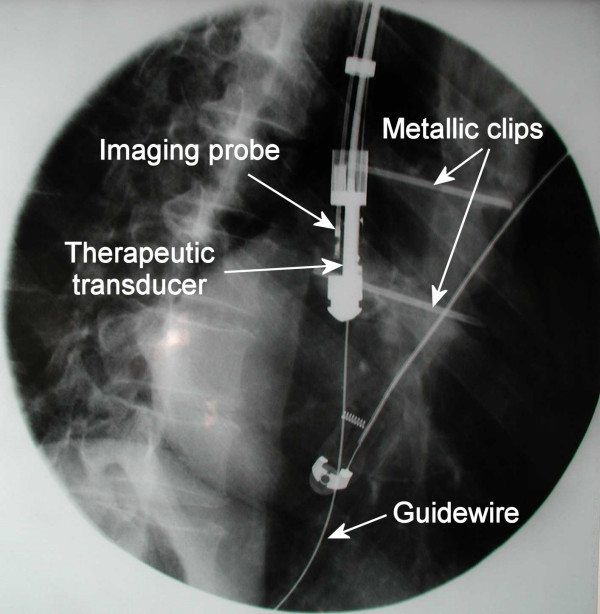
Gross positioning of the therapeutic ultrasound applicator. The therapeutic ultrasound applicator was placed in the esophagus under fluoroscopy after positioning metallic clips on the patient's skin.
